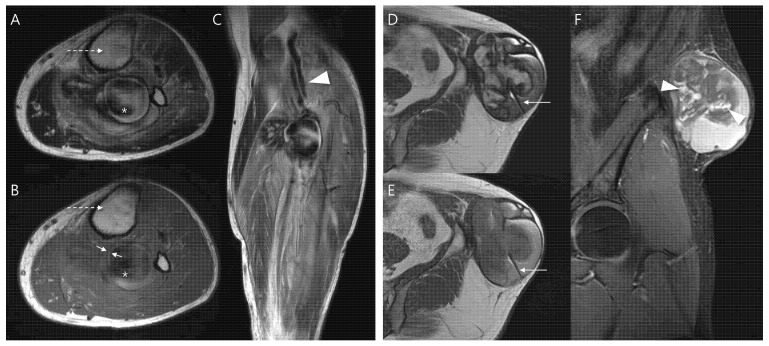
MRI findings of pseudoaneurysm (A–C) and chronic expanding hematoma (D–F). (A) Axial T2-weighted and (B) T1-weighted images show an intramuscular, ovoid mass with a central flow void (asterisk in (A,B), fast-flow blood), pulsatile artifact (dotted arrow in (A,B) and inner peripheral high SI on T1-weighted image (arrows in (B), mural thrombus). (C) Coronal T2-weighted image demonstrates neurovascular involvement (arrowhead). (D) Axial T2-weighted and (E) T1-weighted images reveal a multilobular subcutaneous mass with heterogeneous signal intensity with septations (arrow in (D,E)). (F) Coronal T1-weighted contrast-enhanced image demonstrates nodular enhancement (arrowheads, granulation tissue) within the mass.
